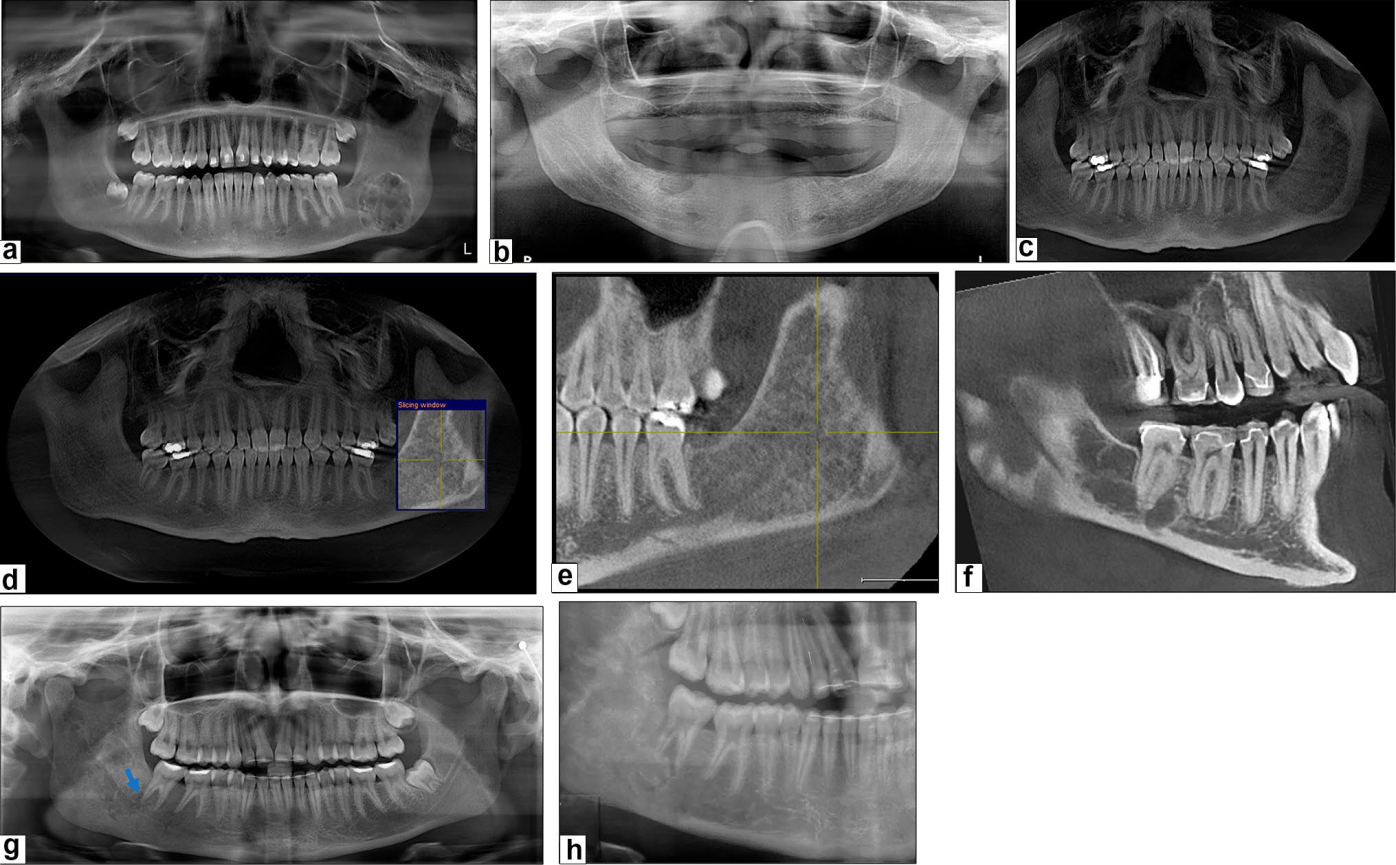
Case 2: a constructed panoramic radiograph shows a well circumscribed mixed radiolucent/radiopaque lesion with sclerotic border. Case 4: b panoramic radiograph demonstrates a unilocular radiolucent lesion at edentulous area corresponding to the site of teeth #28–30. Case 7 c–e: c, constructed panoramic radiograph reveals a well-circumscribed lesion with a ground-glass appearance, involving the left posterior mandible and extending to the ramus. d, e, post-operative follow-up radiographs show evidence of bone regeneration. Case 3: f, CBCT (Sagittal section) showing multilocular radiolucent lesion. Case 1 g–h: g, panoramic radiograph reveals an ill-defined mixed radiolucent/radiopaque lesion with evidence of distal root resorption of tooth #31 (*arrow*). h, post-operative follow-up radiograph showing a residual lesion that appears as an ill-defined radiolucency, with no increase in size or radiodensity
